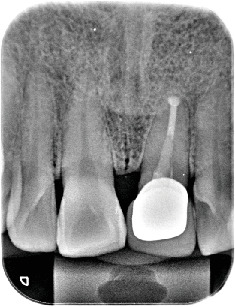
Periapical radiographic evaluation at 55-month follow-up. The radiographs revealed consistent bone density at the apex with adjacent teeth, continuous cortical bone lines on the mesial and distal alveolar crests, and no abnormalities in the periodontal ligament space and no signs of root resorption.
